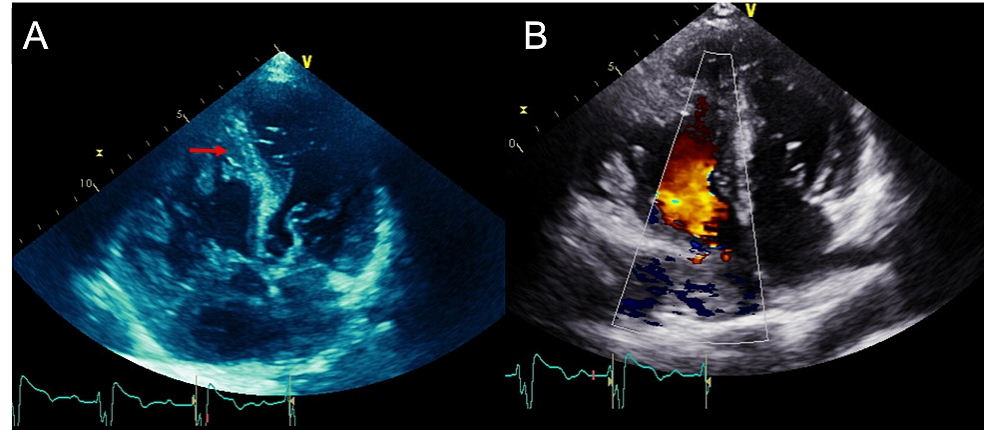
Two-dimensional (A) and color doppler (B) four-chamber views demonstrate a trabeculated right ventricle (arrow).
